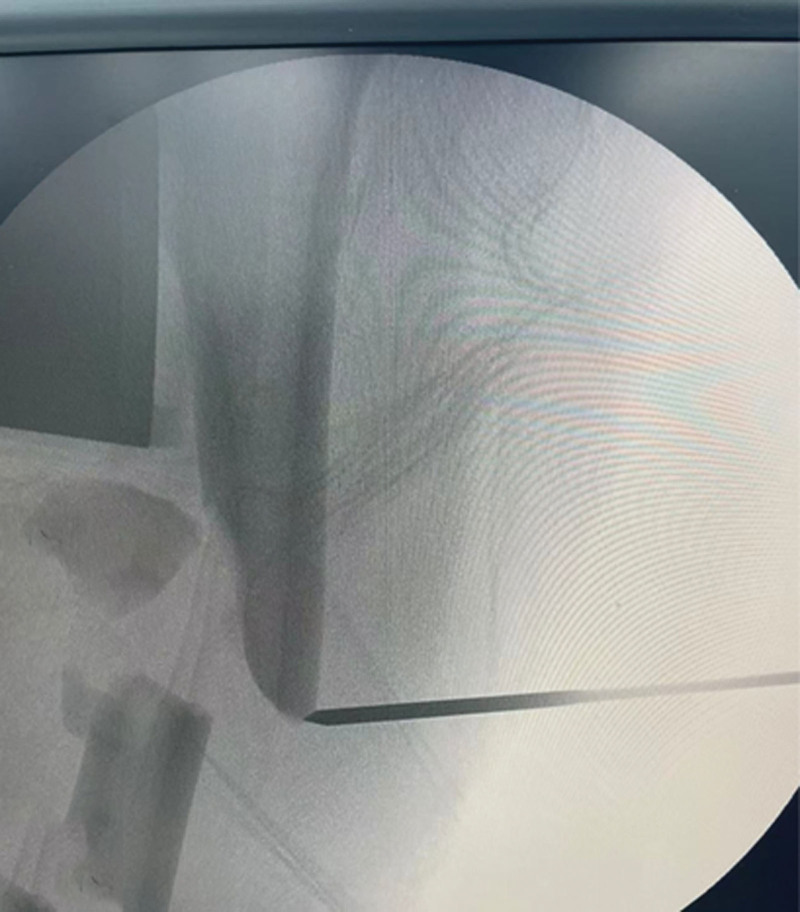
Intraoperative anteroposterior radiograph showed the length recovery after traction, but there was medial-lateral displacement.
